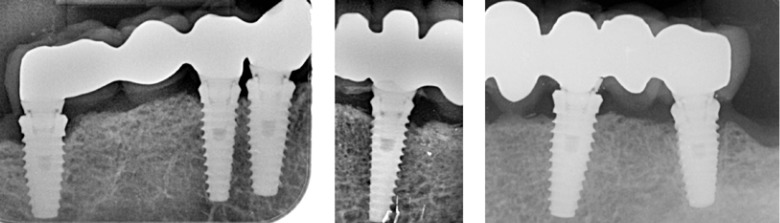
Radiographic assessment at the 12-month follow-up.
